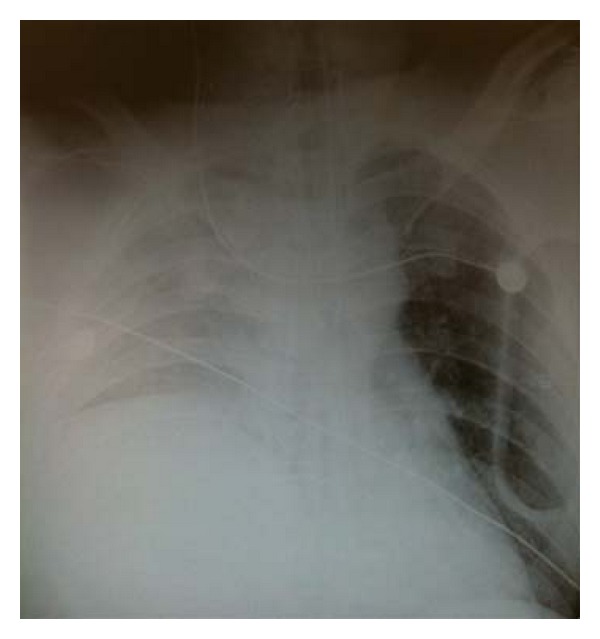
Chest X-ray on arrival to trauma bay.
